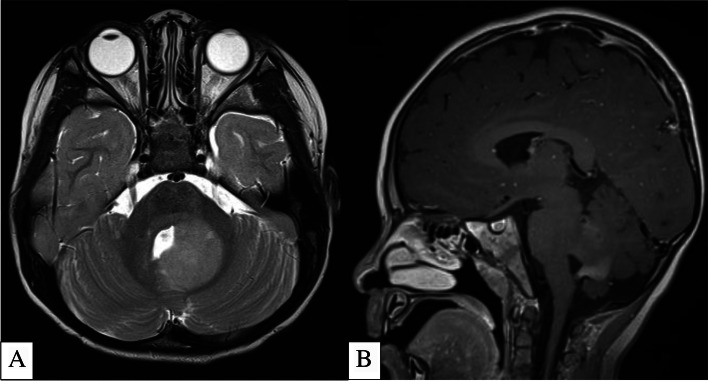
A-B 3-Year-Old Patient with Biopsy Proven Left Cerebellar Ganglioglioma. Axial T2-Weighted MRI (A) shows an expansile, infiltrative, homogenously T2 hyperintense lesion centered at the mesial aspect of the left cerebellar hemisphere and left middle/superior cerebellar peduncles. Sagittal T1-Weighted Post Contrast MRI (B) shows the classic “paintbrush” enhancement within the lesion
